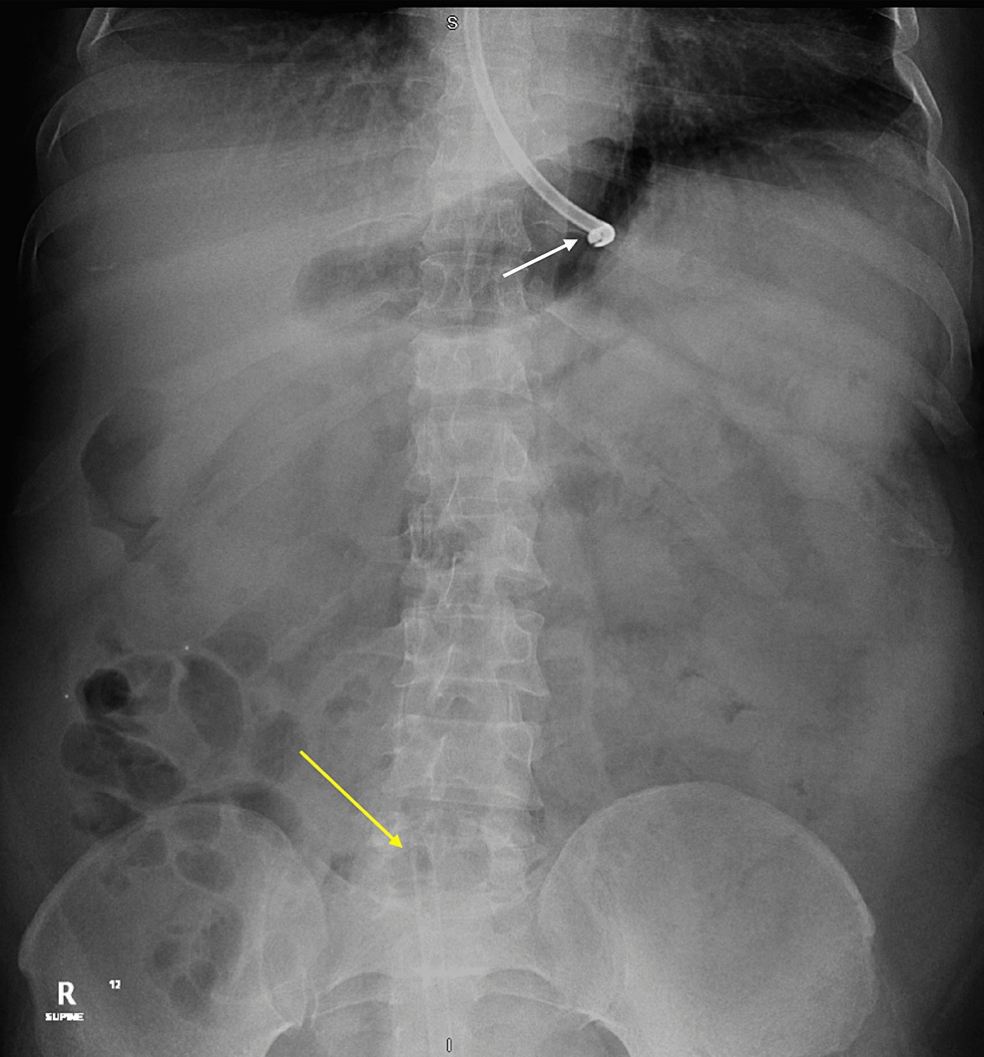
Abdominal x-ray shows resolution of colonic dilatation with Nasogastric tube (White arrow) and Rectal tube (Yellow arrow) in place.
